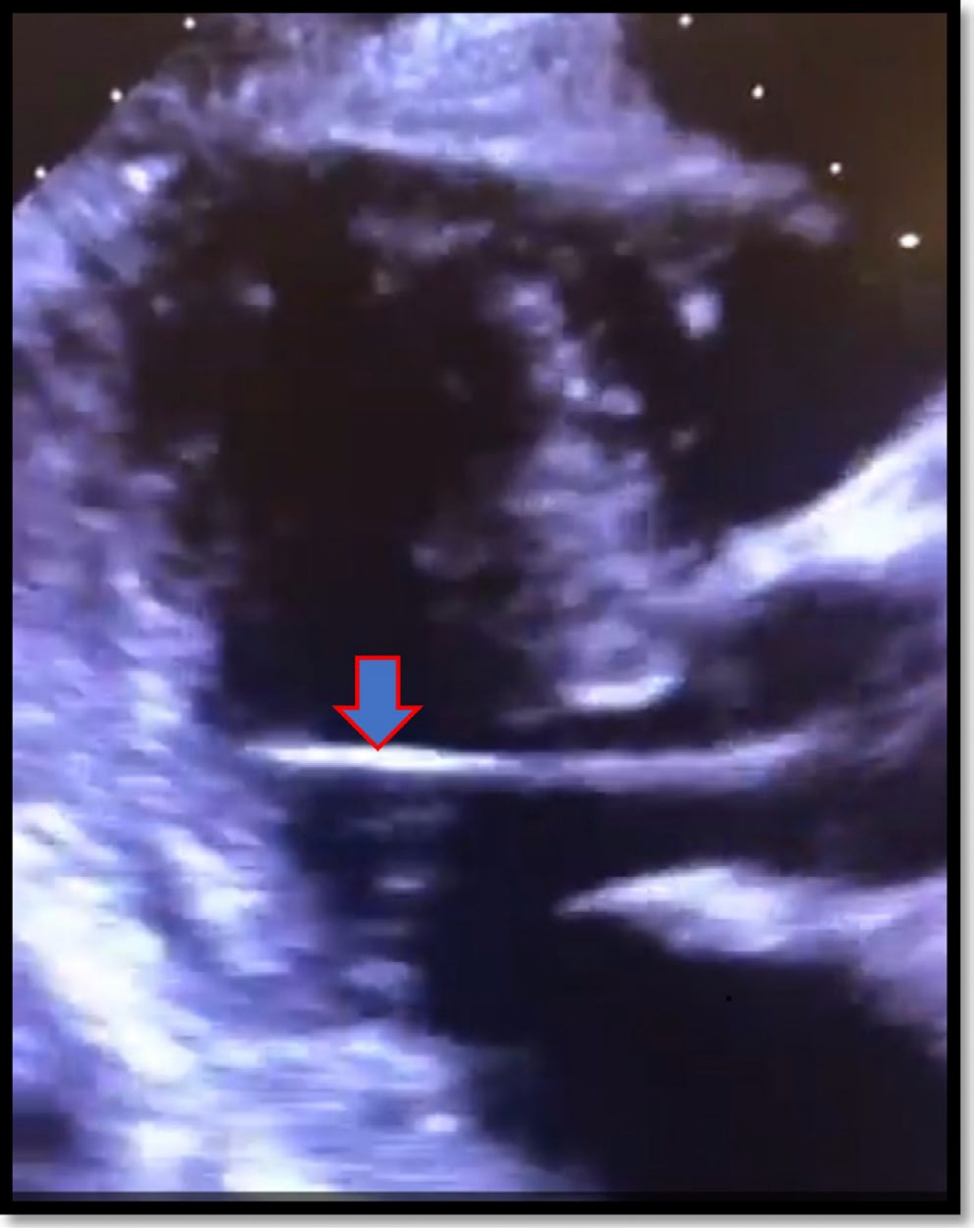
Parasternal long‐axis view showing pacemaker lead (arrow) crossing the aortic valve into the left ventricular cavity and fixed to the inferolateral wall of the left ventricle
